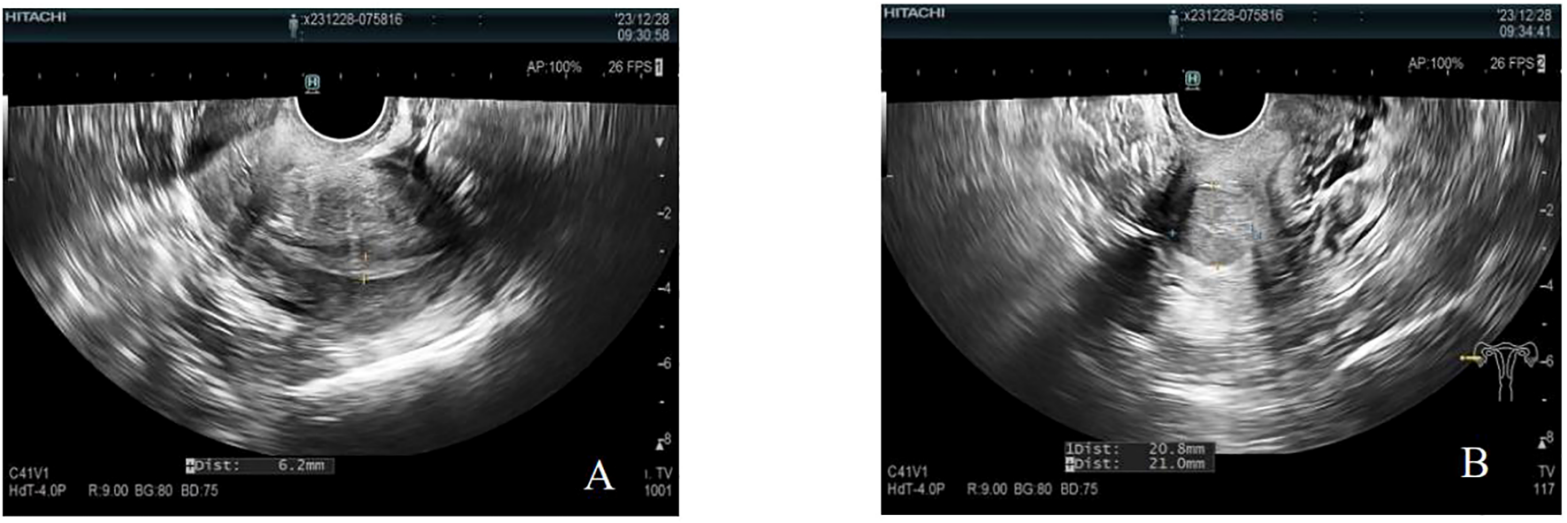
Negative uterus plus double adnexa ultrasound image. (A) The endometrium was clear, centered, 6 mm thick, with uneven echogenicity. (B) A hypoechoic area was seen in the cervix, approximately 21 × 21 mm in size, with poorly defined borders and poorly homogenized internal echogenicity. CDFI showed no obvious blood flow signal was seen in and around the hypoechoic area of the cervix.
